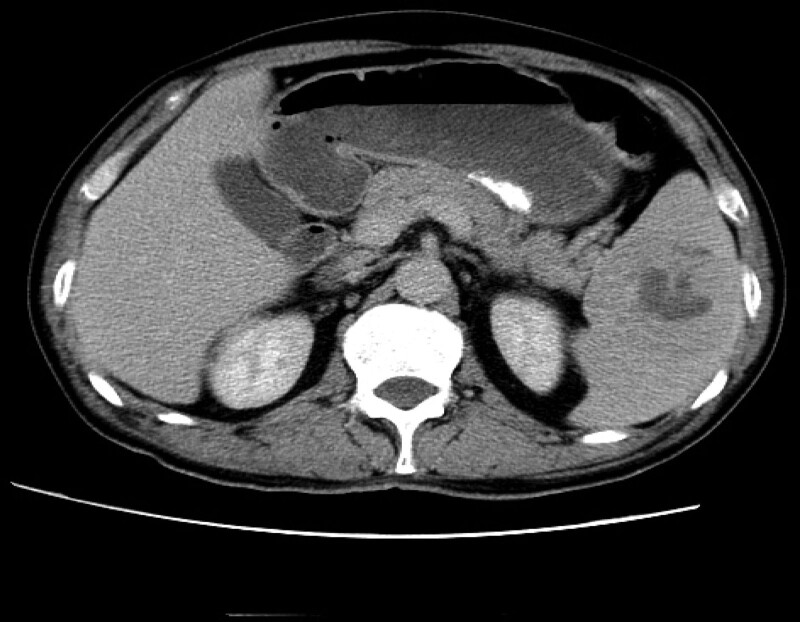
CT imaging before surgery. spleen mass and intrahepatic nodule, considering spleen tumor with intrahepatic metastasis.
